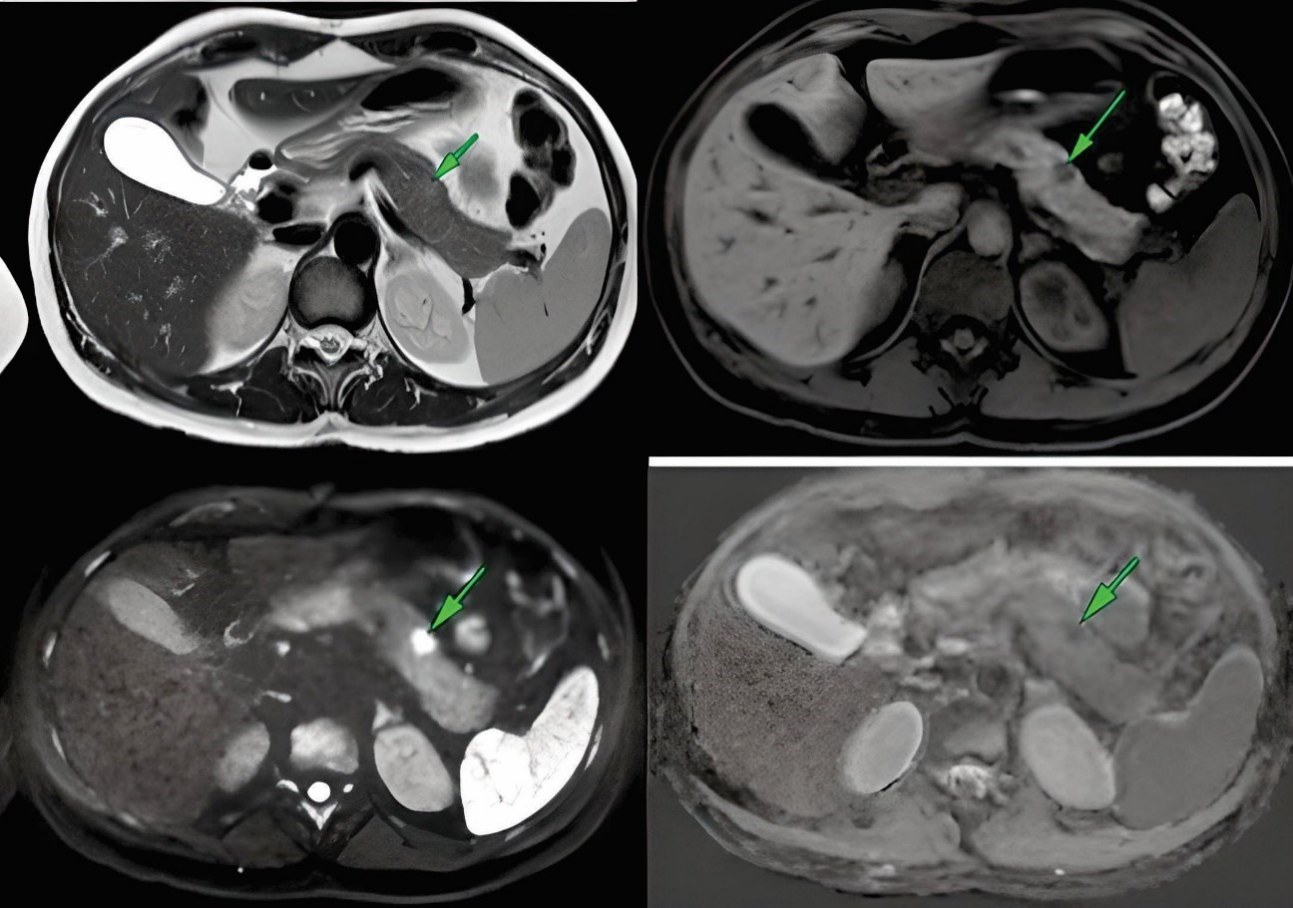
Preoperative magnetic resonance imaging.
